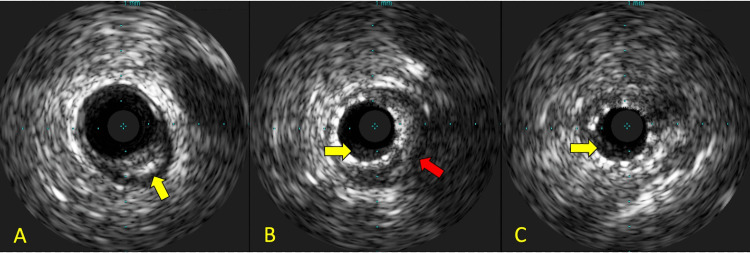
IVUS after achieving revascularization. (A) Segment immediately proximal to stent implantation demonstrates atheromatous plaque (yellow arrow). (B) The proximal stent segment demonstrates in-stent restenosis and residual thrombus within (yellow arrow) and behind the stent (red arrow). (C) Distal stent segment with stenosis and residual thrombus within the stent (yellow arrow). IVUS: intravascular ultrasound.
